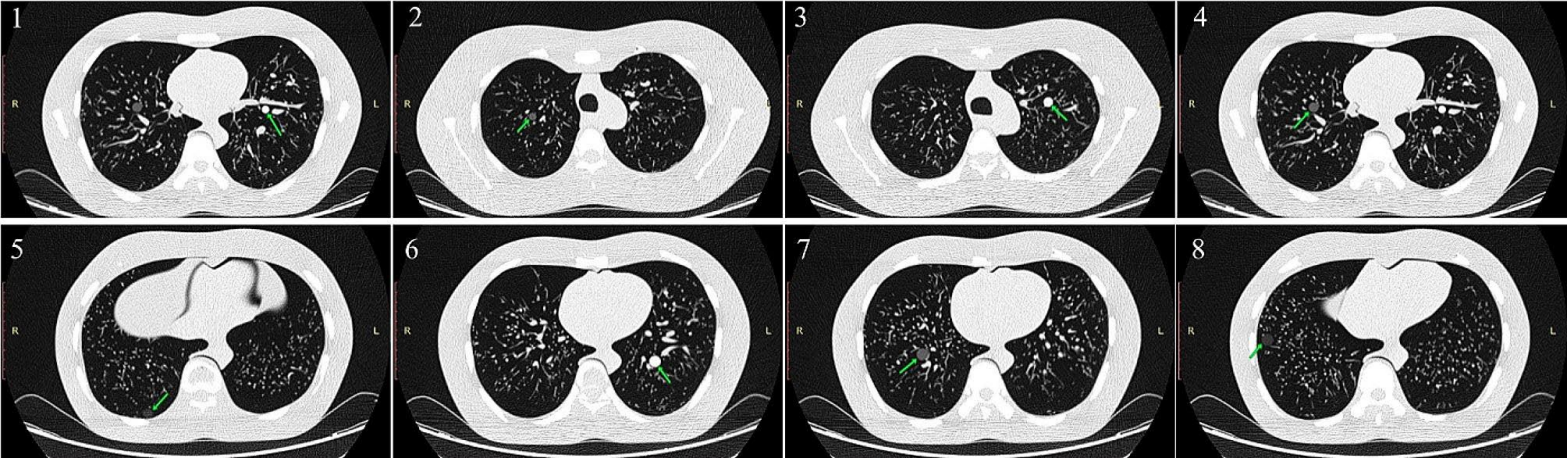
The LUNGMAN N1 model CT image. Nodule #1 is 8 mm, 100HU, nodule #2 is 8 mm, − 630HU, nodule #3 is 10 mm, 100HU, nodule #4 is 10 mm, − 630HU, nodule #5 is 10 mm, − 800HU, nodule #6 is 12 mm, 100HU, nodule #7 is 12 mm, − 630HU, nodule #8 is 12 mm, − 800HU.
